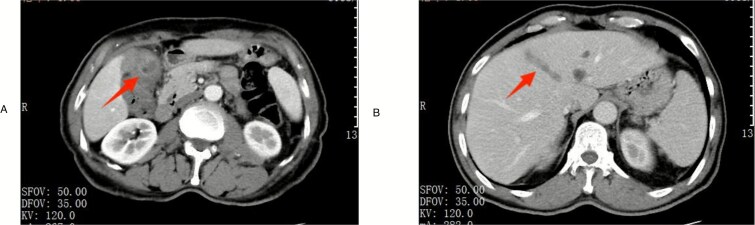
Preoperative imaging. (A) Contrast-enhanced CT scan in the arterial phase demonstrating gallbladder wall thickening with enhancement (arrow). (B) Contrast-enhanced CT in the venous phase showing thrombus formation within the middle hepatic vein (arrowhead).
